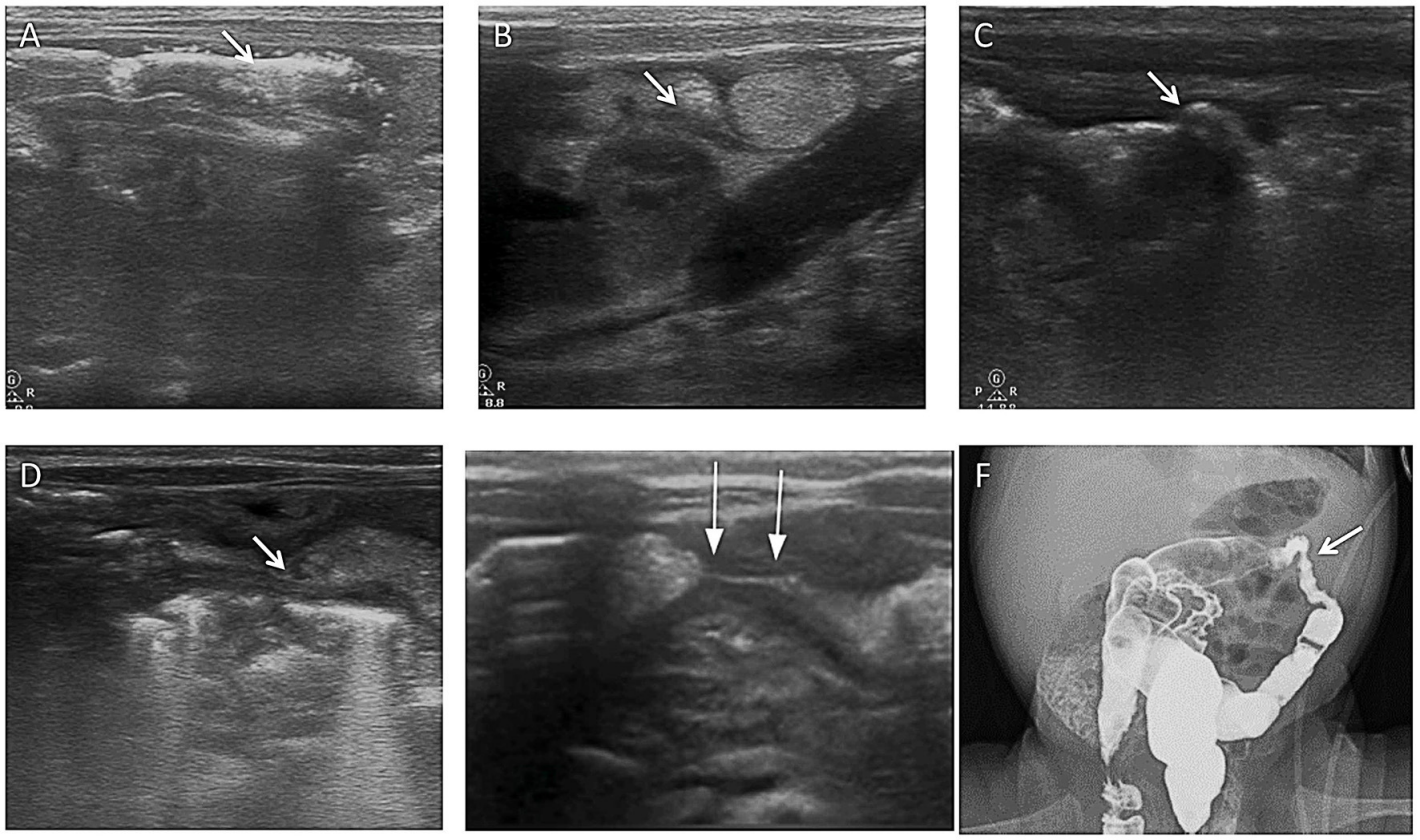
Images show a post-NEC intestinal stricture evaluated by AUS. (A) Multiple mural gases were seen in the transverse colon on the 1st day of NEC (arrow). (B) After 11 days, the wall of the transverse colon was irregularly thickened (arrow). (C) On the 24th day, the wall of the transverse colon was irregularly thickened, and the intestinal lumen was stiff and irregular (arrow). (D) On the 38th day, the wall of the transverse colon was irregularly thickened, the shape of the intestinal lumen was rigid, and the intestinal lumen was narrow (arrow). (E) On the 61st day, the wall of the transverse colon was irregularly thickened, the shape of the intestinal lumen was rigid, and significant stenosis of the intestinal lumen was seen (arrow). (F) On the 61st day, total gastrointestinal radiography with Iohexol showed severe stenosis in the colon, proving the result by AUS (arrow).
